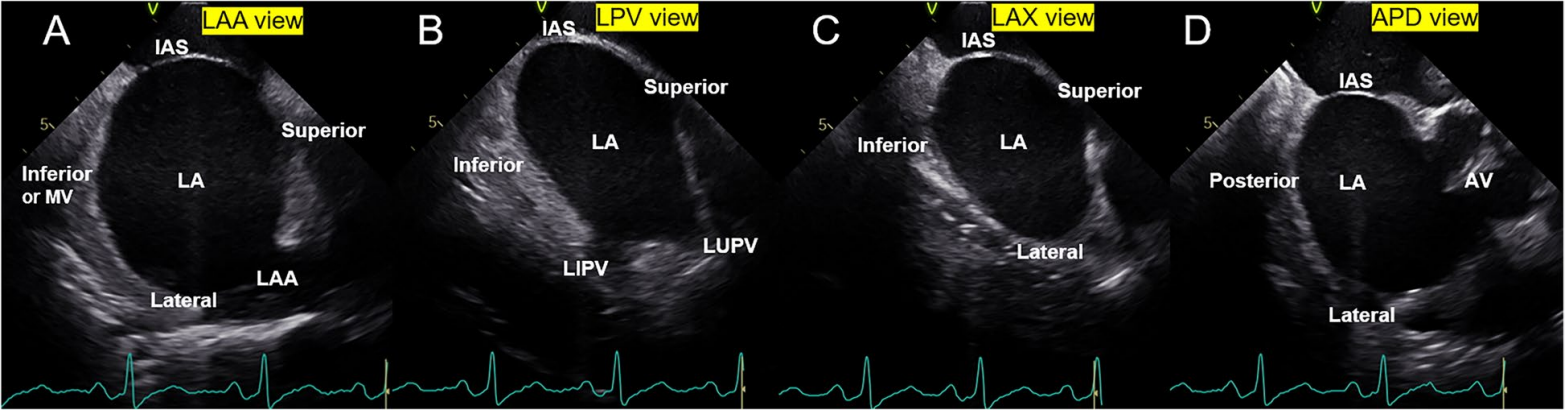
LA images derived from ICE. (A) LA appendage (LAA) view image; (B) LA left pulmonary vein (LPV) view image; (C) LA Long-axis (LAX) view image; (D) LA anterior–posterior dimension (APD) view image; AV, aortic valve; IAS, interatrial septum; ICE, intracardiac echocardiography; LA, left atrial; LAA, left atrial appendage; LIPV, left inferior pulmonary vein; LUPV, left superior pulmonary vein; MV, mitral valve
